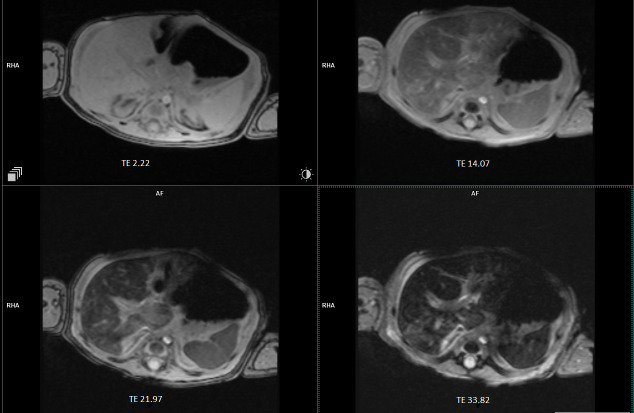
Abdominal MRI showing the decreased signal intensity in the liver parenchyma with no extrahepatic siderosis. MRI: magnetic resonance imaging.
